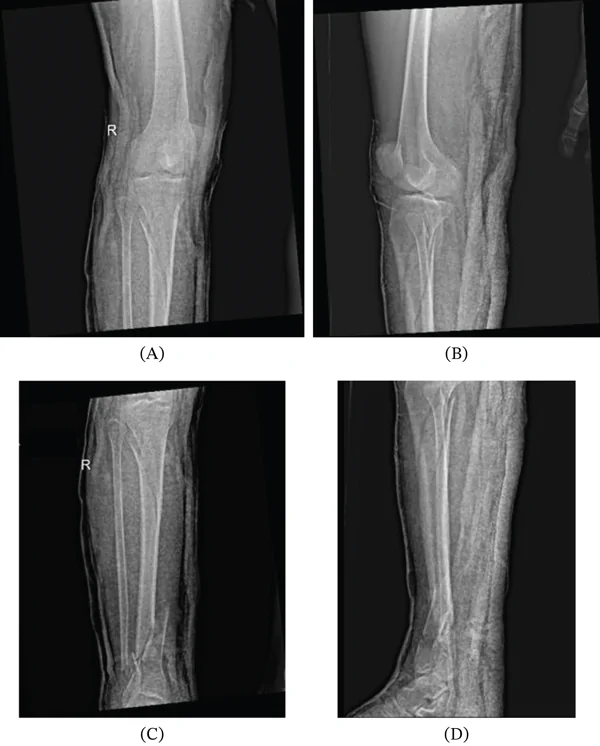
Preoperative plain radiographs. (A) Anteroposterior knee view and (B) lateral knee view demonstrate the bicondylar proximal tibial injury. (C) Anteroposterior distal‐leg/ankle view and (D) lateral distal‐leg/ankle view demonstrate the distal tibial plafond and associated fibular fractures. CT was obtained preoperatively but the original CT image files are no longer retrievable because of archival and storage limitations described in the text.
